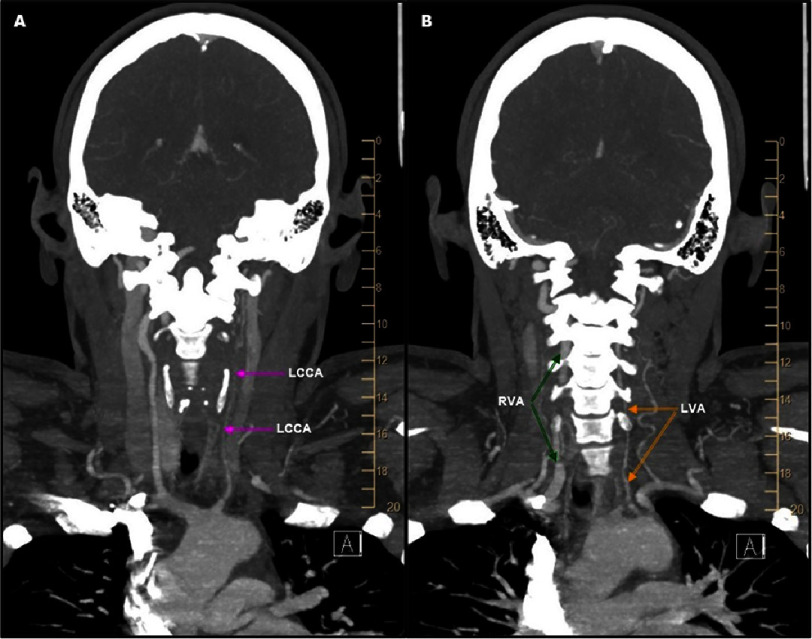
Computed tomography angiogram of head and neck. (A) LCCA with stenosis (pink arrows). (B) Hypoplastic LVA (orange arrows) and compensatory hypertrophic right vertebral artery (RVA) (dark green arrows).
